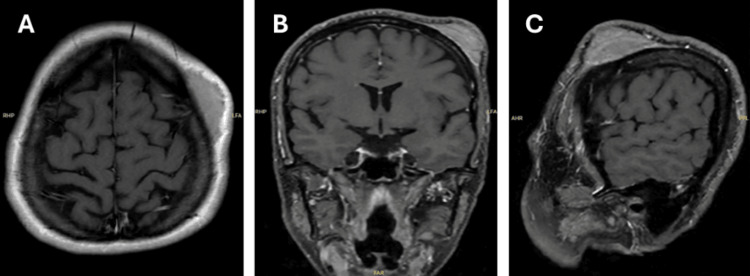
(A-C) Preoperative MRI T1 images after gadolinium injection (axial, coronal, and sagittal, respectively) showing the scalp lesion. Note: No bone or dural enhancement.
